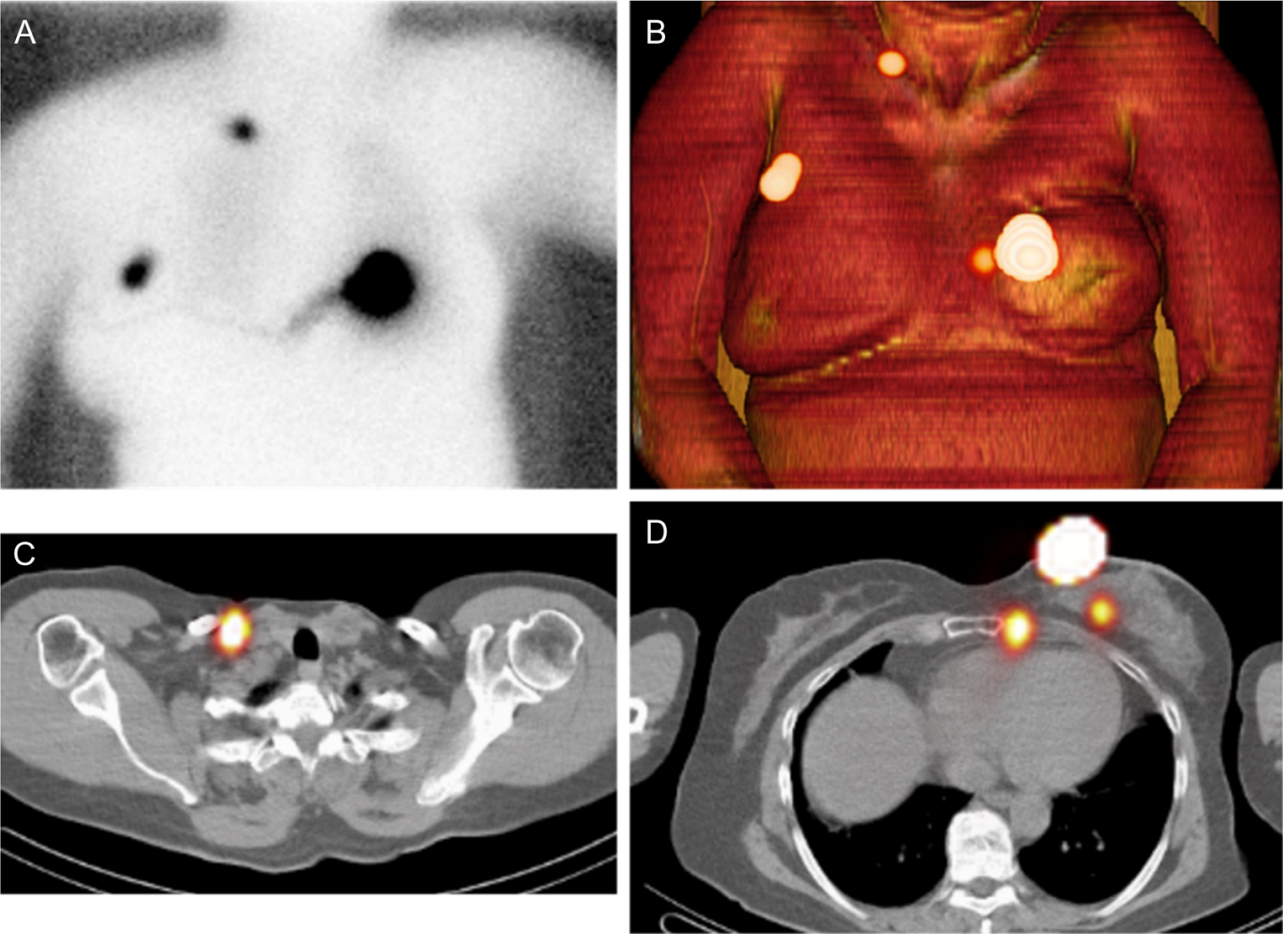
A 61-year-old woman with ductal carcinoma relapse in the left breast. (a) Late planar scintigraphy shows a lymphatic duct towards the contralateral axilla. Also, a supraclavicular SN is visualized. (b) Volume rendering shows both axillary and supraclavicular SNs together with a focus medial to the injection site and altered anatomical configuration of the left breast area due to primary treatment. (c) Supraclavicular SN on the right. (d) Dorsal of the injection site two SN are visualized on SPECT/CT, one intramammary and one intercostal, not visualized on planar scintigraphy. Histopatology demonstrated micrometastasis in the axillary node whereas the intramammary, intercostal and supraclavicular SN were negative
